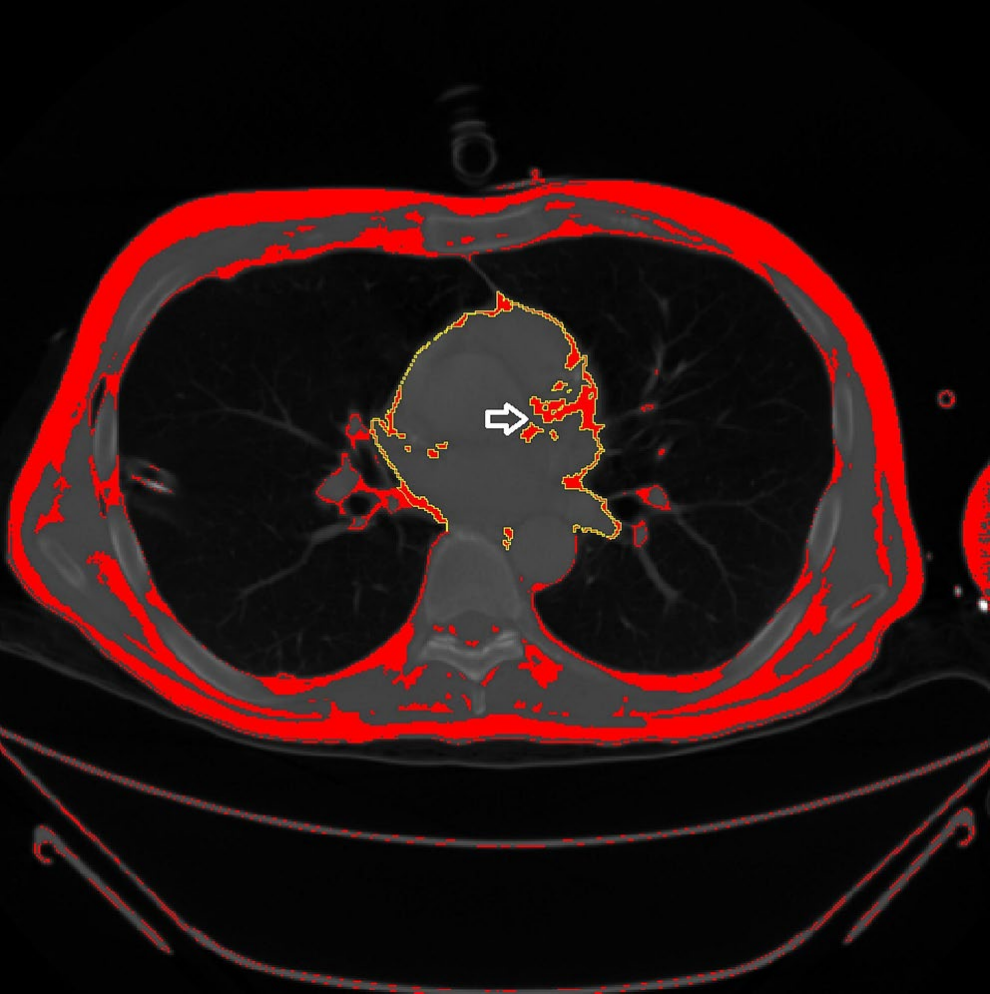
Representative 49-years old male patient of the patient cohort with an ISS score of 29. Axial CT slice with the measured EAT highlighted demonstrated in yellow. The arrow indicates the origin of the left main coronary artery
